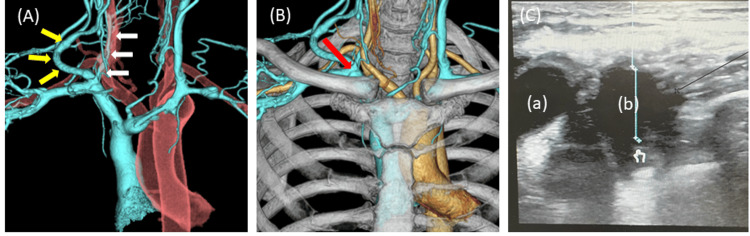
Computed tomography (CT) image taken before the dual-lumen cannula (DLC) insertion (A) A merged CT venography (CTV) and CT angiography (CTA) image of the jugular and thoracic veins and arteries. The yellow arrows indicate a large, well-developed, right external jugular vein functioning as a collateral vessel. The white arrows indicate the right internal jugular vein stenosed by a thrombus. (B) Integration of CTV, CTA, and bones. The red arrow indicates the site where the DLC was inserted. (C) Ultrasound image of the right supraclavicular approach. The right external jugular vein root (a) and the right subclavian vein root (b) are shown. The distance from the skin to the anterior wall of the punctured vessel was only 1 cm.
